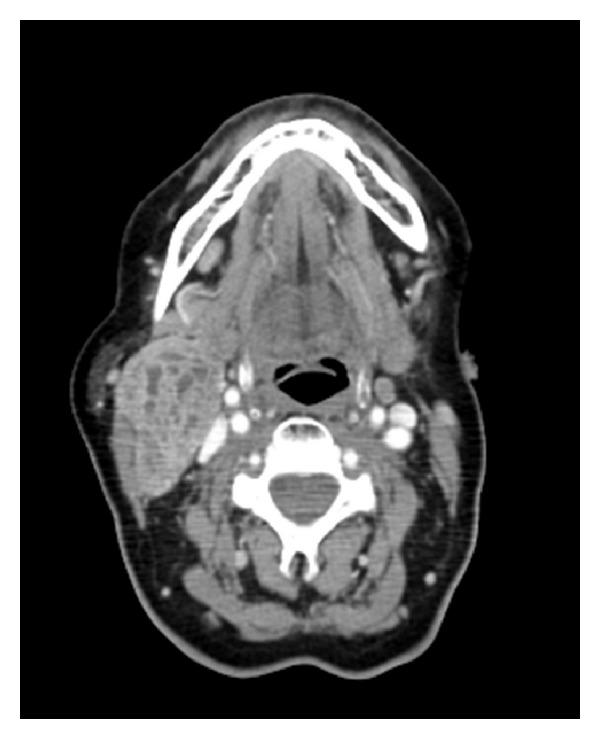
The neoplasm in lateral cervical region (maximum length of 6 cm) confirmed by CT scan.
